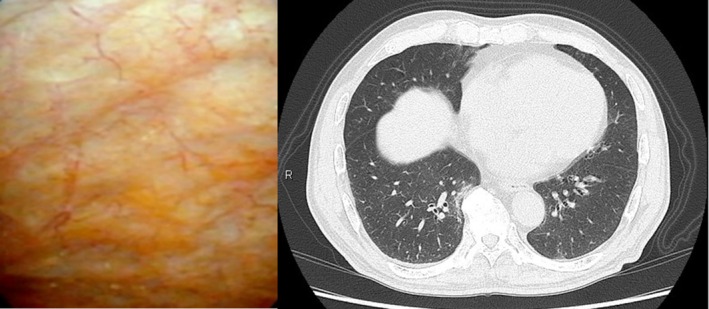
Cystoscopy and chest CT at 5 months post‐surgery. The tumors have disappeared.
